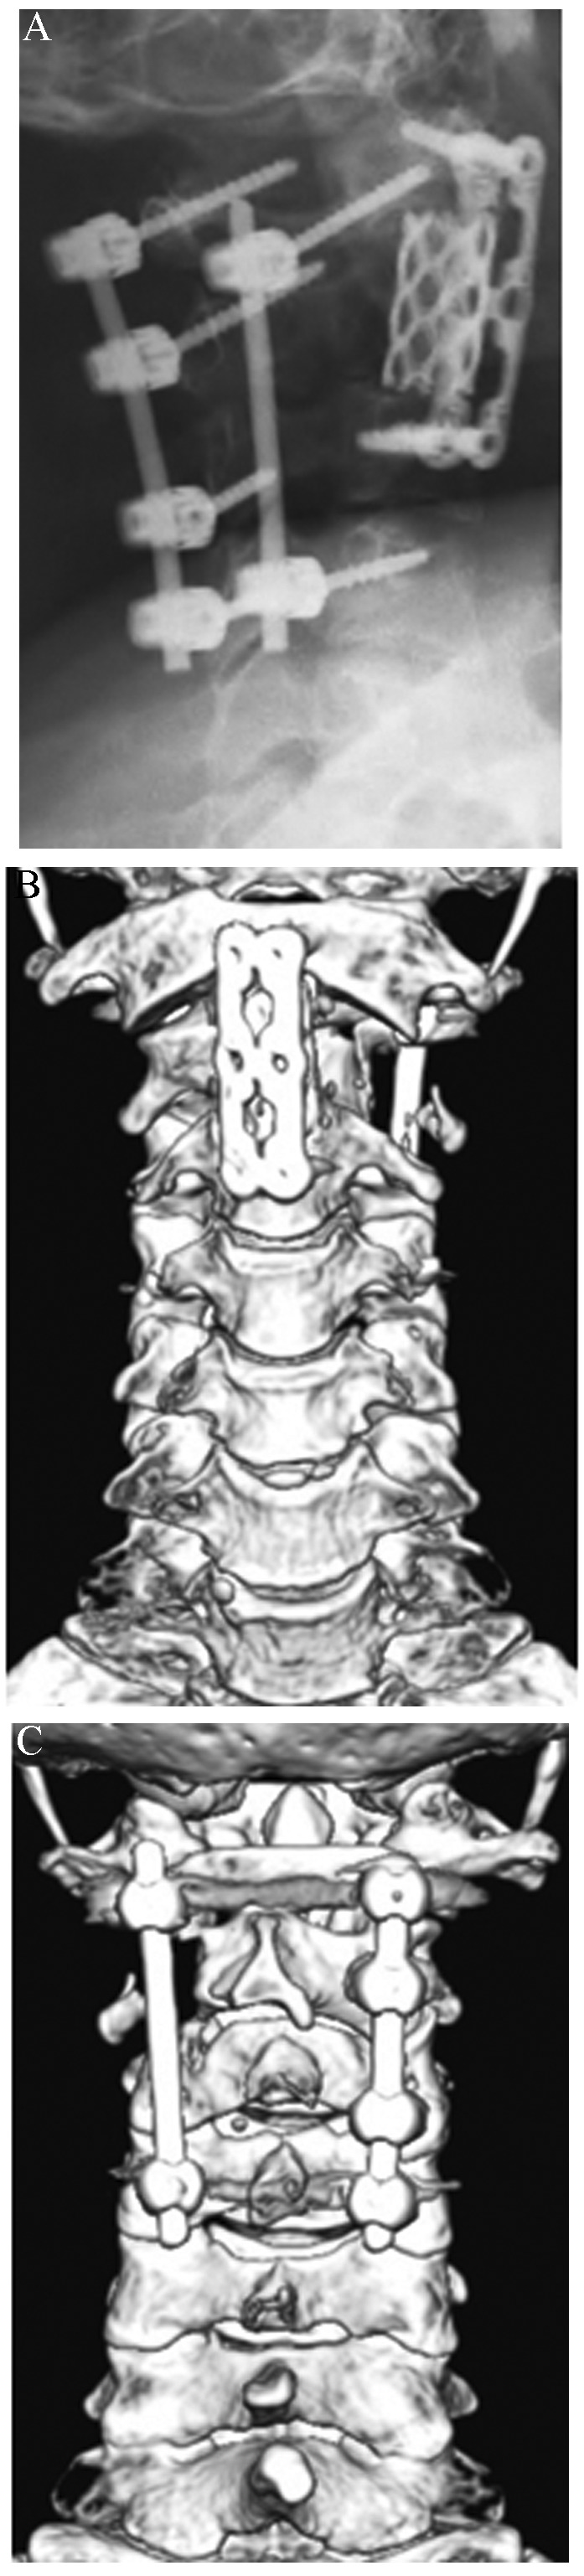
Postoperative images at the six month follow up showed no signs of implant dislocation. (A) Lateral X-ray. (B and C) CT 3D. CT, computed tomography; 3D, three dimensional.
